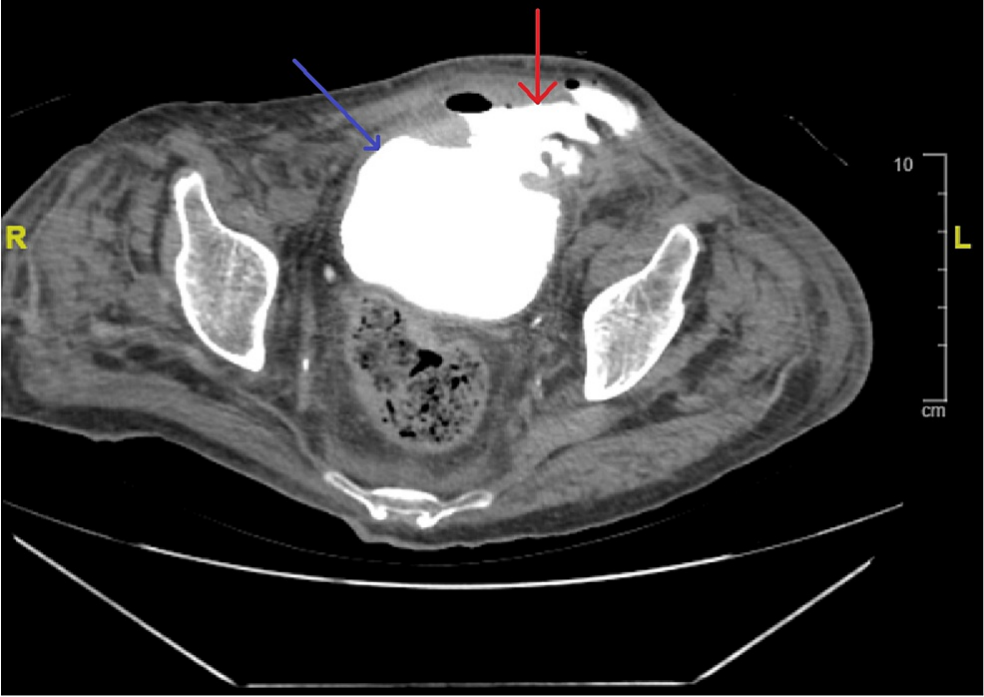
Computed tomography (axial view) shows a urinary bladder (blue arrow) anterior superior wall large defect, measuring about 4.3×4 cm, communicating with the anterior pelvic encysted fluid collection (red arrow).
